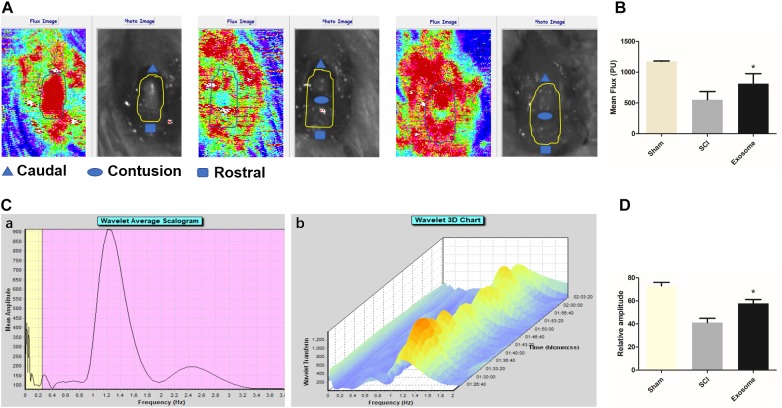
Pericytes exosomes promoted blood flow and improved endothelial function after SCI. (A) The blood flow of organs was detected by the Laser Doppler Line Scanner. The video image and flux image of spinal cord in the three groups: Sham, SCI, exosomes. The area of interest is circled by a yellow curve. (B) Quantification of blood flow in the three groups. (C) The vasomotion of spinal cord was measured by dual channel laser doppler monitor, and the LDF signal was analyzed by wavelet analysis. The wavelet analysis was performed by means of the Moor software. Corresponding vasomotor amplitude during study period (a). A three-dimensional (3D) plot was produced from the wavelet transformations of perfusion signals (b). (D) The values of the relative amplitudes of oscillatory LDF signal in the endothelial factor. *n* = 5 in each group. ^∗^*p* < 0.05 compared with SCI group.
